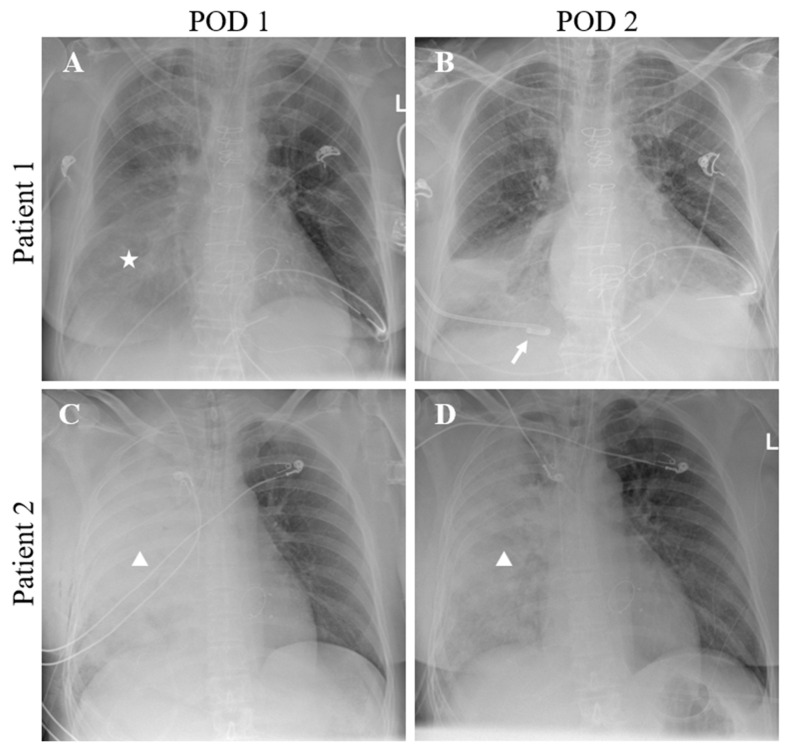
Two different clinical situations after MVS with unilateral opacities on chest X-ray. The first postoperative chest X-ray (A) of a patient after MVS shows unilateral opacities of the right hemithorax (star), which resolves after insertion of a pleural drain (Arrow, (B)). Therefore, the cause of the opacities can be attributed to pleural effusion with atelectasis rather than UPE. In another patient, chest X-ray on the first (C) and second day (D) after surgery showed a persisting consolidated unilateral opacity of the right hemithorax (triangles), suggesting UPE.
